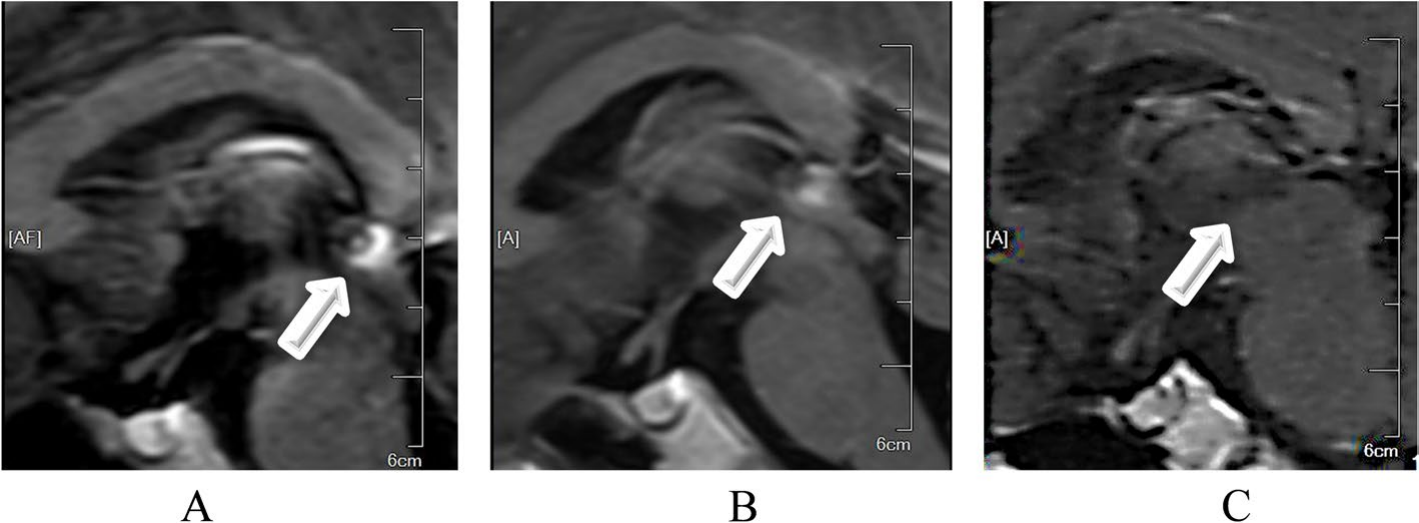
Contrast-enhanced brain MRI. (A) Sagittal contrast-enhanced brain MRI at diagnosis, (B) sagittal contrast-enhanced brain MRI after 2 courses of chemotherapy, (C) sagittal contrast-enhanced brain MRI after chemoradiotherapy with additional neurosurgical operation.1A showed a homogenously enhancing pineal mass (marked in white arrow); 1B showed the similar tumor imaging (marked in white arrow) to 1A although a mild shrinkage; 1C showed a disorder of pineal structure (marked in white arrow)
